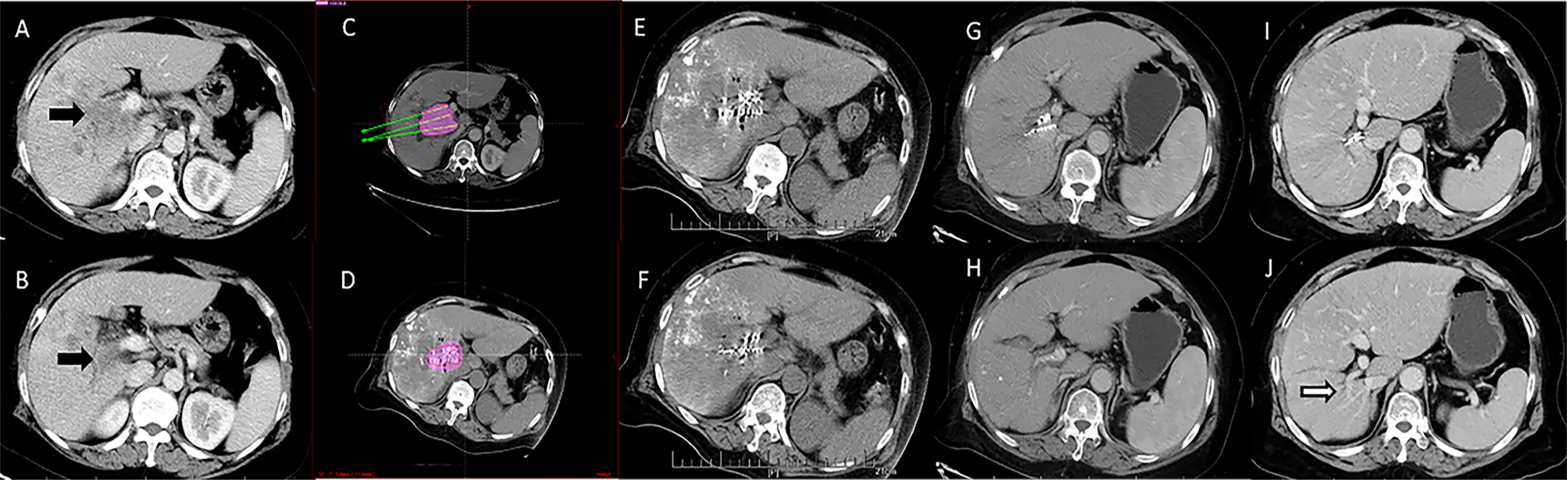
Computed tomography imaging of a 67-year-old woman with hepatocellular carcinoma complicated by portal vein tumor thrombosis in the right branch (Type IIa). (A, B) Enhanced computed tomography imaging showing a portal vein tumor thrombus originating from the right branch of the portal vein. The image before iodine seed implantation showed (C) The spatial distribution of seeds according to the treatment planning system (TPS), and (D) dosage verification, with good results according to the TPS. (E, F) Computed tomography imaging demonstrated that the iodine seed was distributed in the PVTT, the PVTT had decreased in size, and (G, H) the portal vein was partly recanalized at 3 months after seed implantation. (I, J) The portal vein was completely recanalized at 12 months after the procedure, and the patient remained alive until the end of follow-up.
